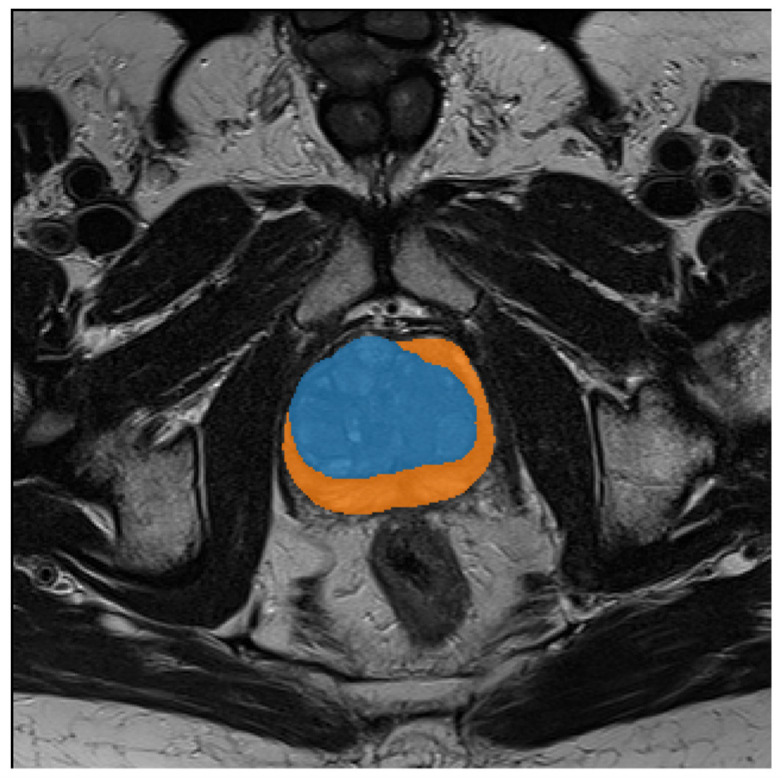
Visualization of the manual annotations prepared for each prostate MRI scan. The inner regions are depicted in blue, while orange shows the outer prostate region.
